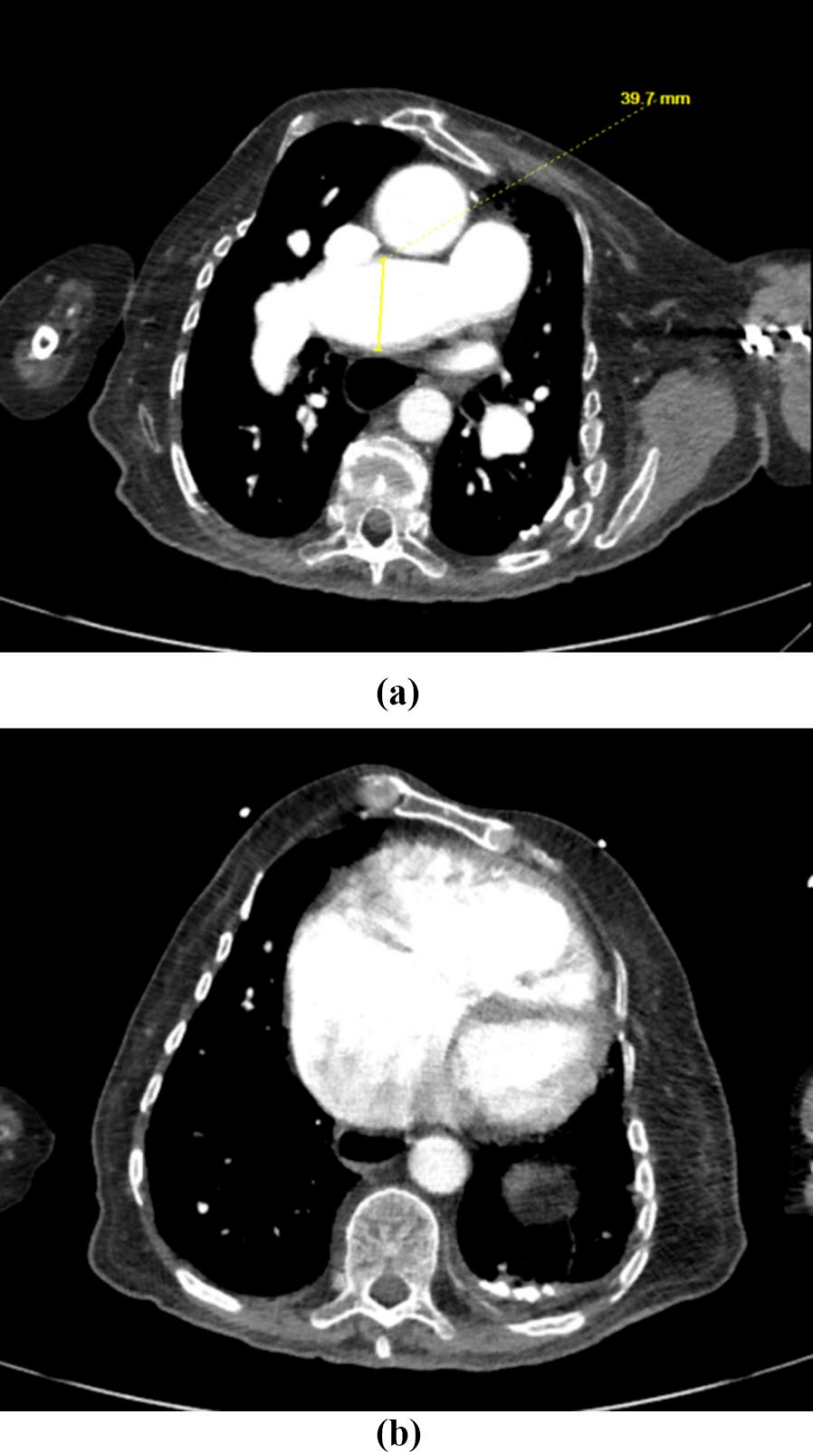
CT scan showing severe pulmonary arterial dilatation indicative of pulmonary hypertension (a) with severe RV dilatation (b)
